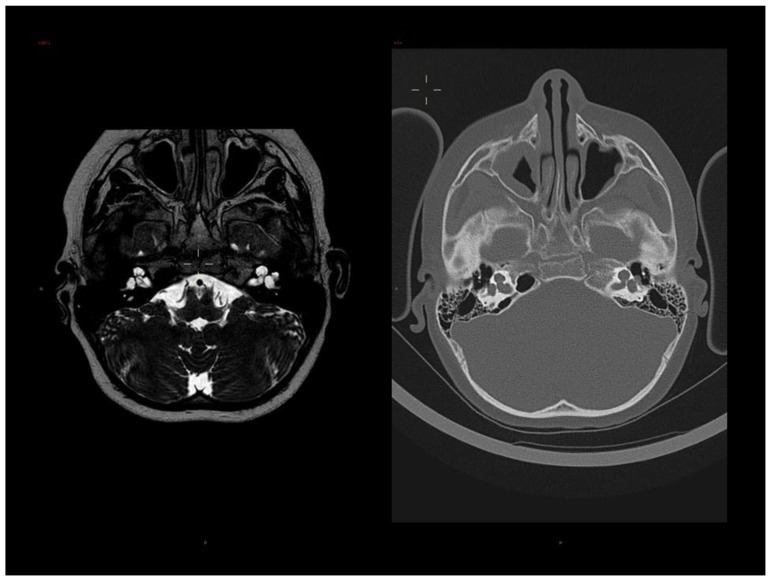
Brain MRI and CT of the proband, showing bilateral incomplete partition type I with defective internal structure of the cochlea and mildly enlarged vestibule on both sides, especially on the left, where a focal herniation of perilymphatic fluid through discontinuity of the bone wall at the stapedo-ovalar junction is observed. Mild enlargement of the right vestibular acqueduct and of the lateral semicircular canals.
